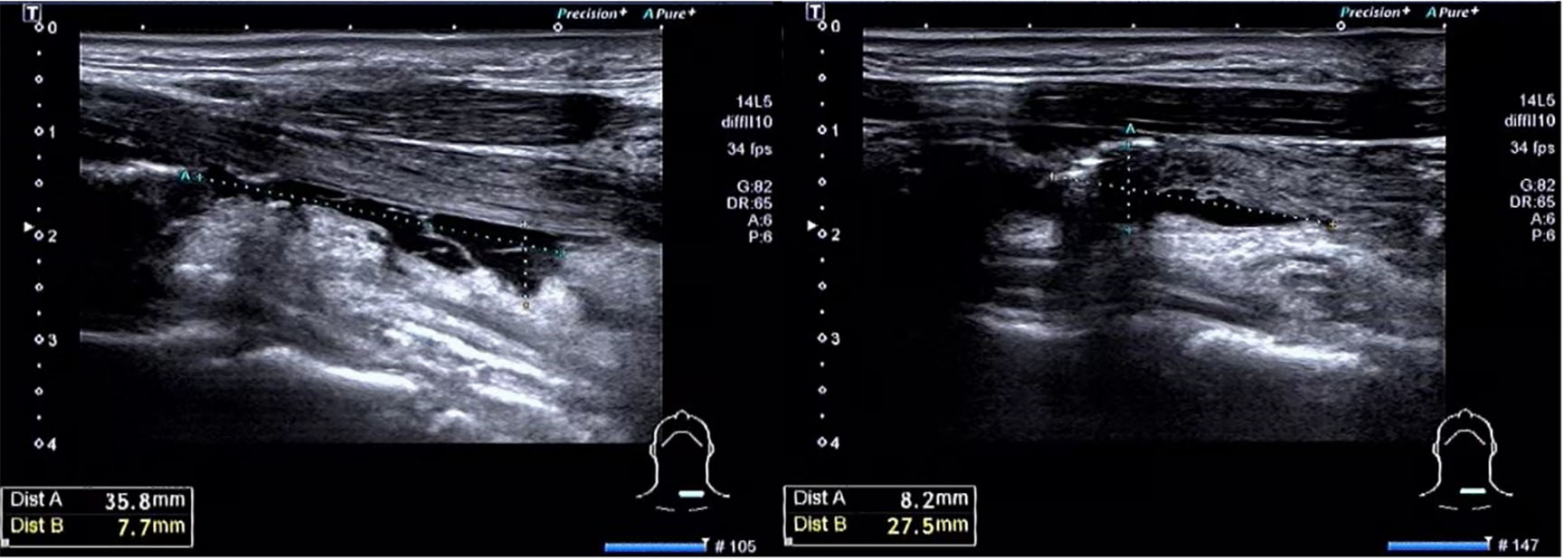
Measurement of cervical effusion by ultrasound.
